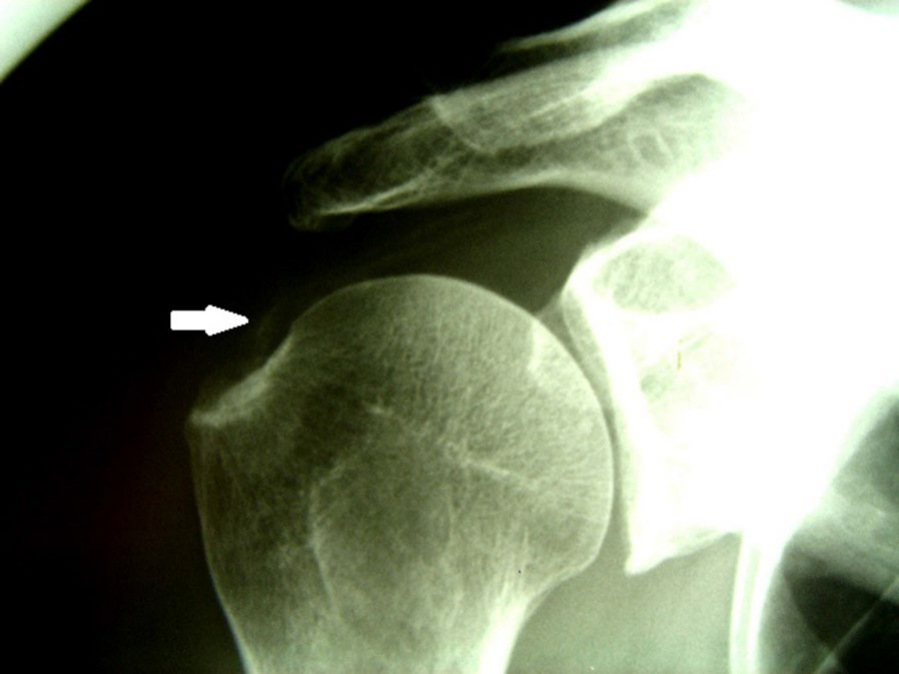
AP X-ray of the shoulder showing a type III calcifying lesion. *White arrow* pointing at the soft contour of the linear calcifications infiltrating the tendon
